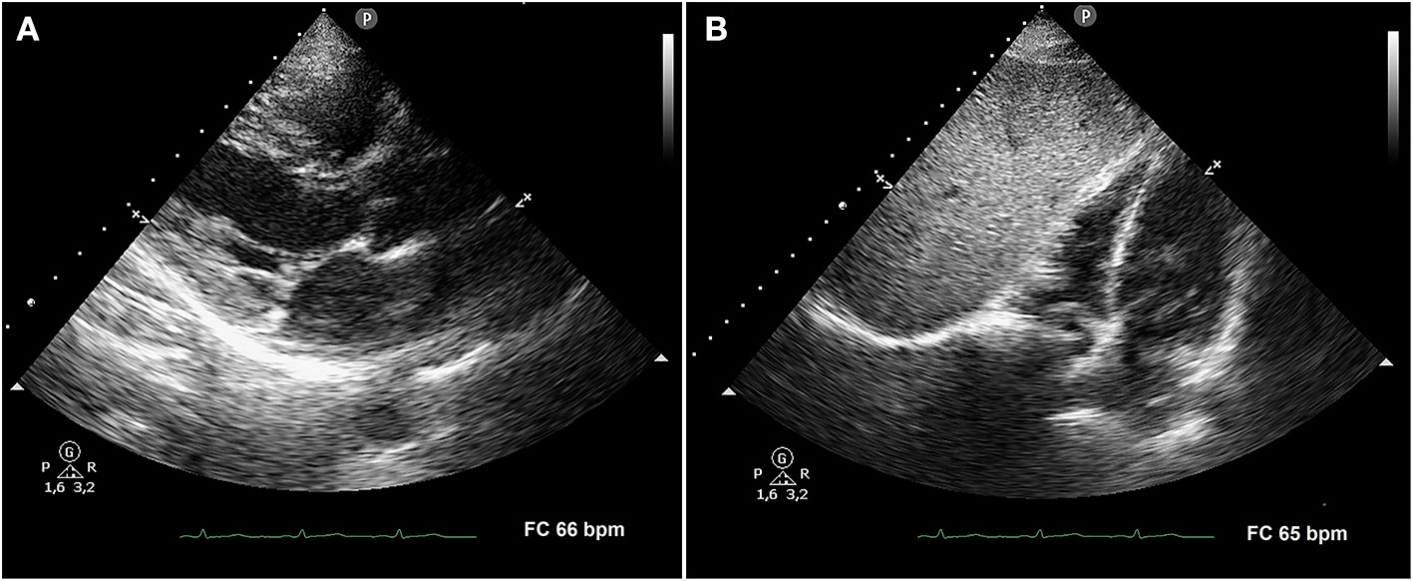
Transthoracic echocardiography performed after 4 weeks of anti-inflammatory treatment. Parasternal long-axis view (A) and subcostal four-chamber view (B) demonstrating the complete disappearance of both posterior pericardial detachment and anterior pericardial effusion, respectively.
